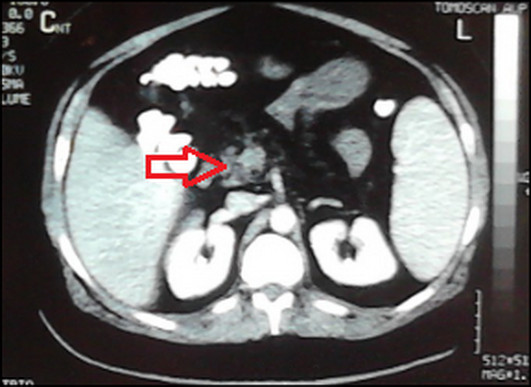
Abdominal computed tomographic scan showing the presence of a thrombus about 7 mm in diameter at the proximal superior mesenteric vein.
